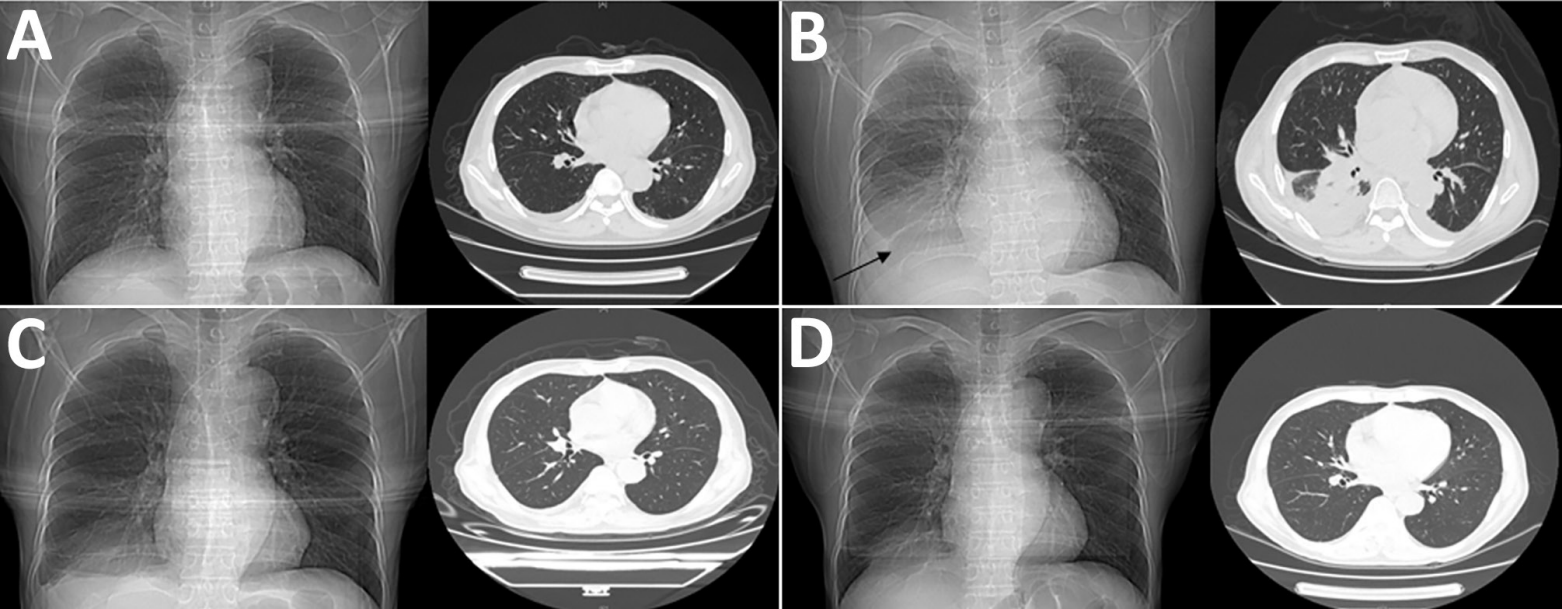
Chest radiography and computed tomography images during acute infection with *Legionella pneumophila* subspecies *fraseri* after allogeneic hematopoietic stem cell transplant, China, 2021. A) May 13 (day 104), B) May 17 (day 108), C) May 24 (day 115), D) June 1 (day 123). Arrow in panel B indicates a large, solid, fuzzy shadow with unclear boundary, uneven internal density, and low-density plaques in the lower lobe of the right lung.
